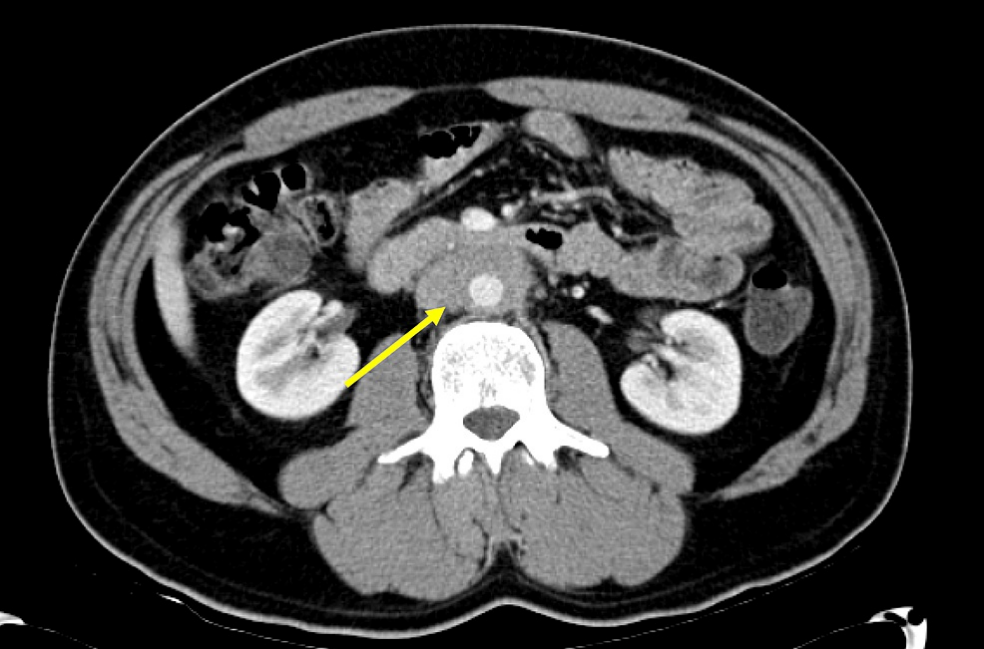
Repeat CT at the time of diagnosis. Circumferential soft tissue thickening around the distal abdominal aorta and proximal right common iliac measuring up to 1 cm in thickness (arrow). There is no appreciable hydronephrosis or ureteral displacement.
